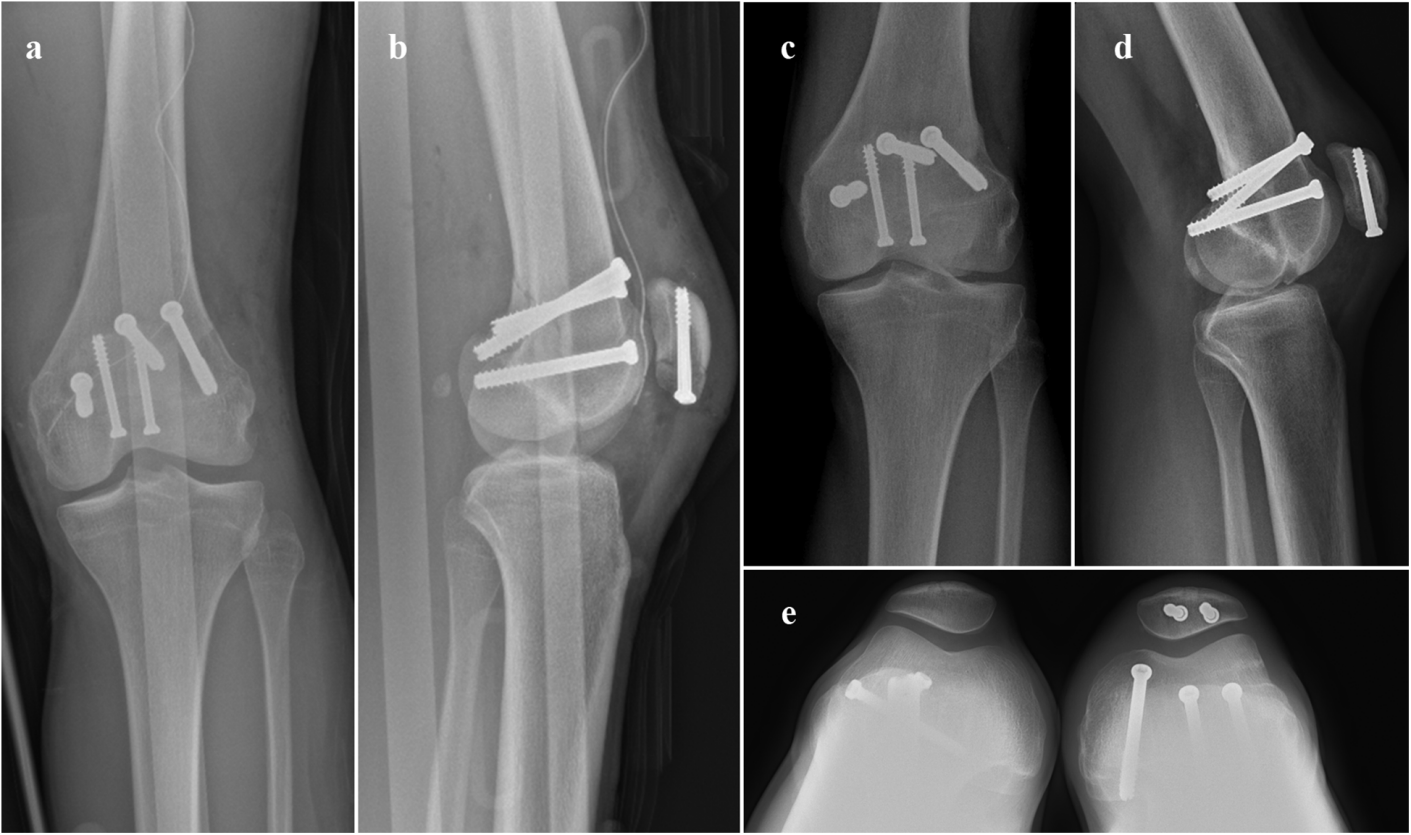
Immediate postoperative x-rays examination AP (a) and lateral view (b); and postoperative x-rays at 6 months follow-up AP (c), lateral (d) and patellofemoral view (e).
